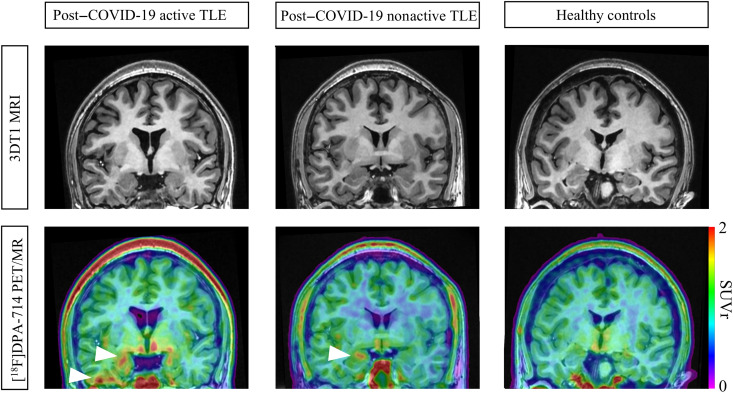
[^18^F]DPA-714 PET/MR of patients with TLE and HCs. 3DT1-MRI (top), [^18^F]DPA-714 PET/MR (bottom) images of three representative participants [one case of a patient with post–COVID-19 active TLE (left), one case of a patient with post–COVID-19 nonactive TLE (middle), and one case of an HC (right)]. Focal uptake in the hippocampus/temporal lobe of the affected hemisphere is marked with an arrow.
